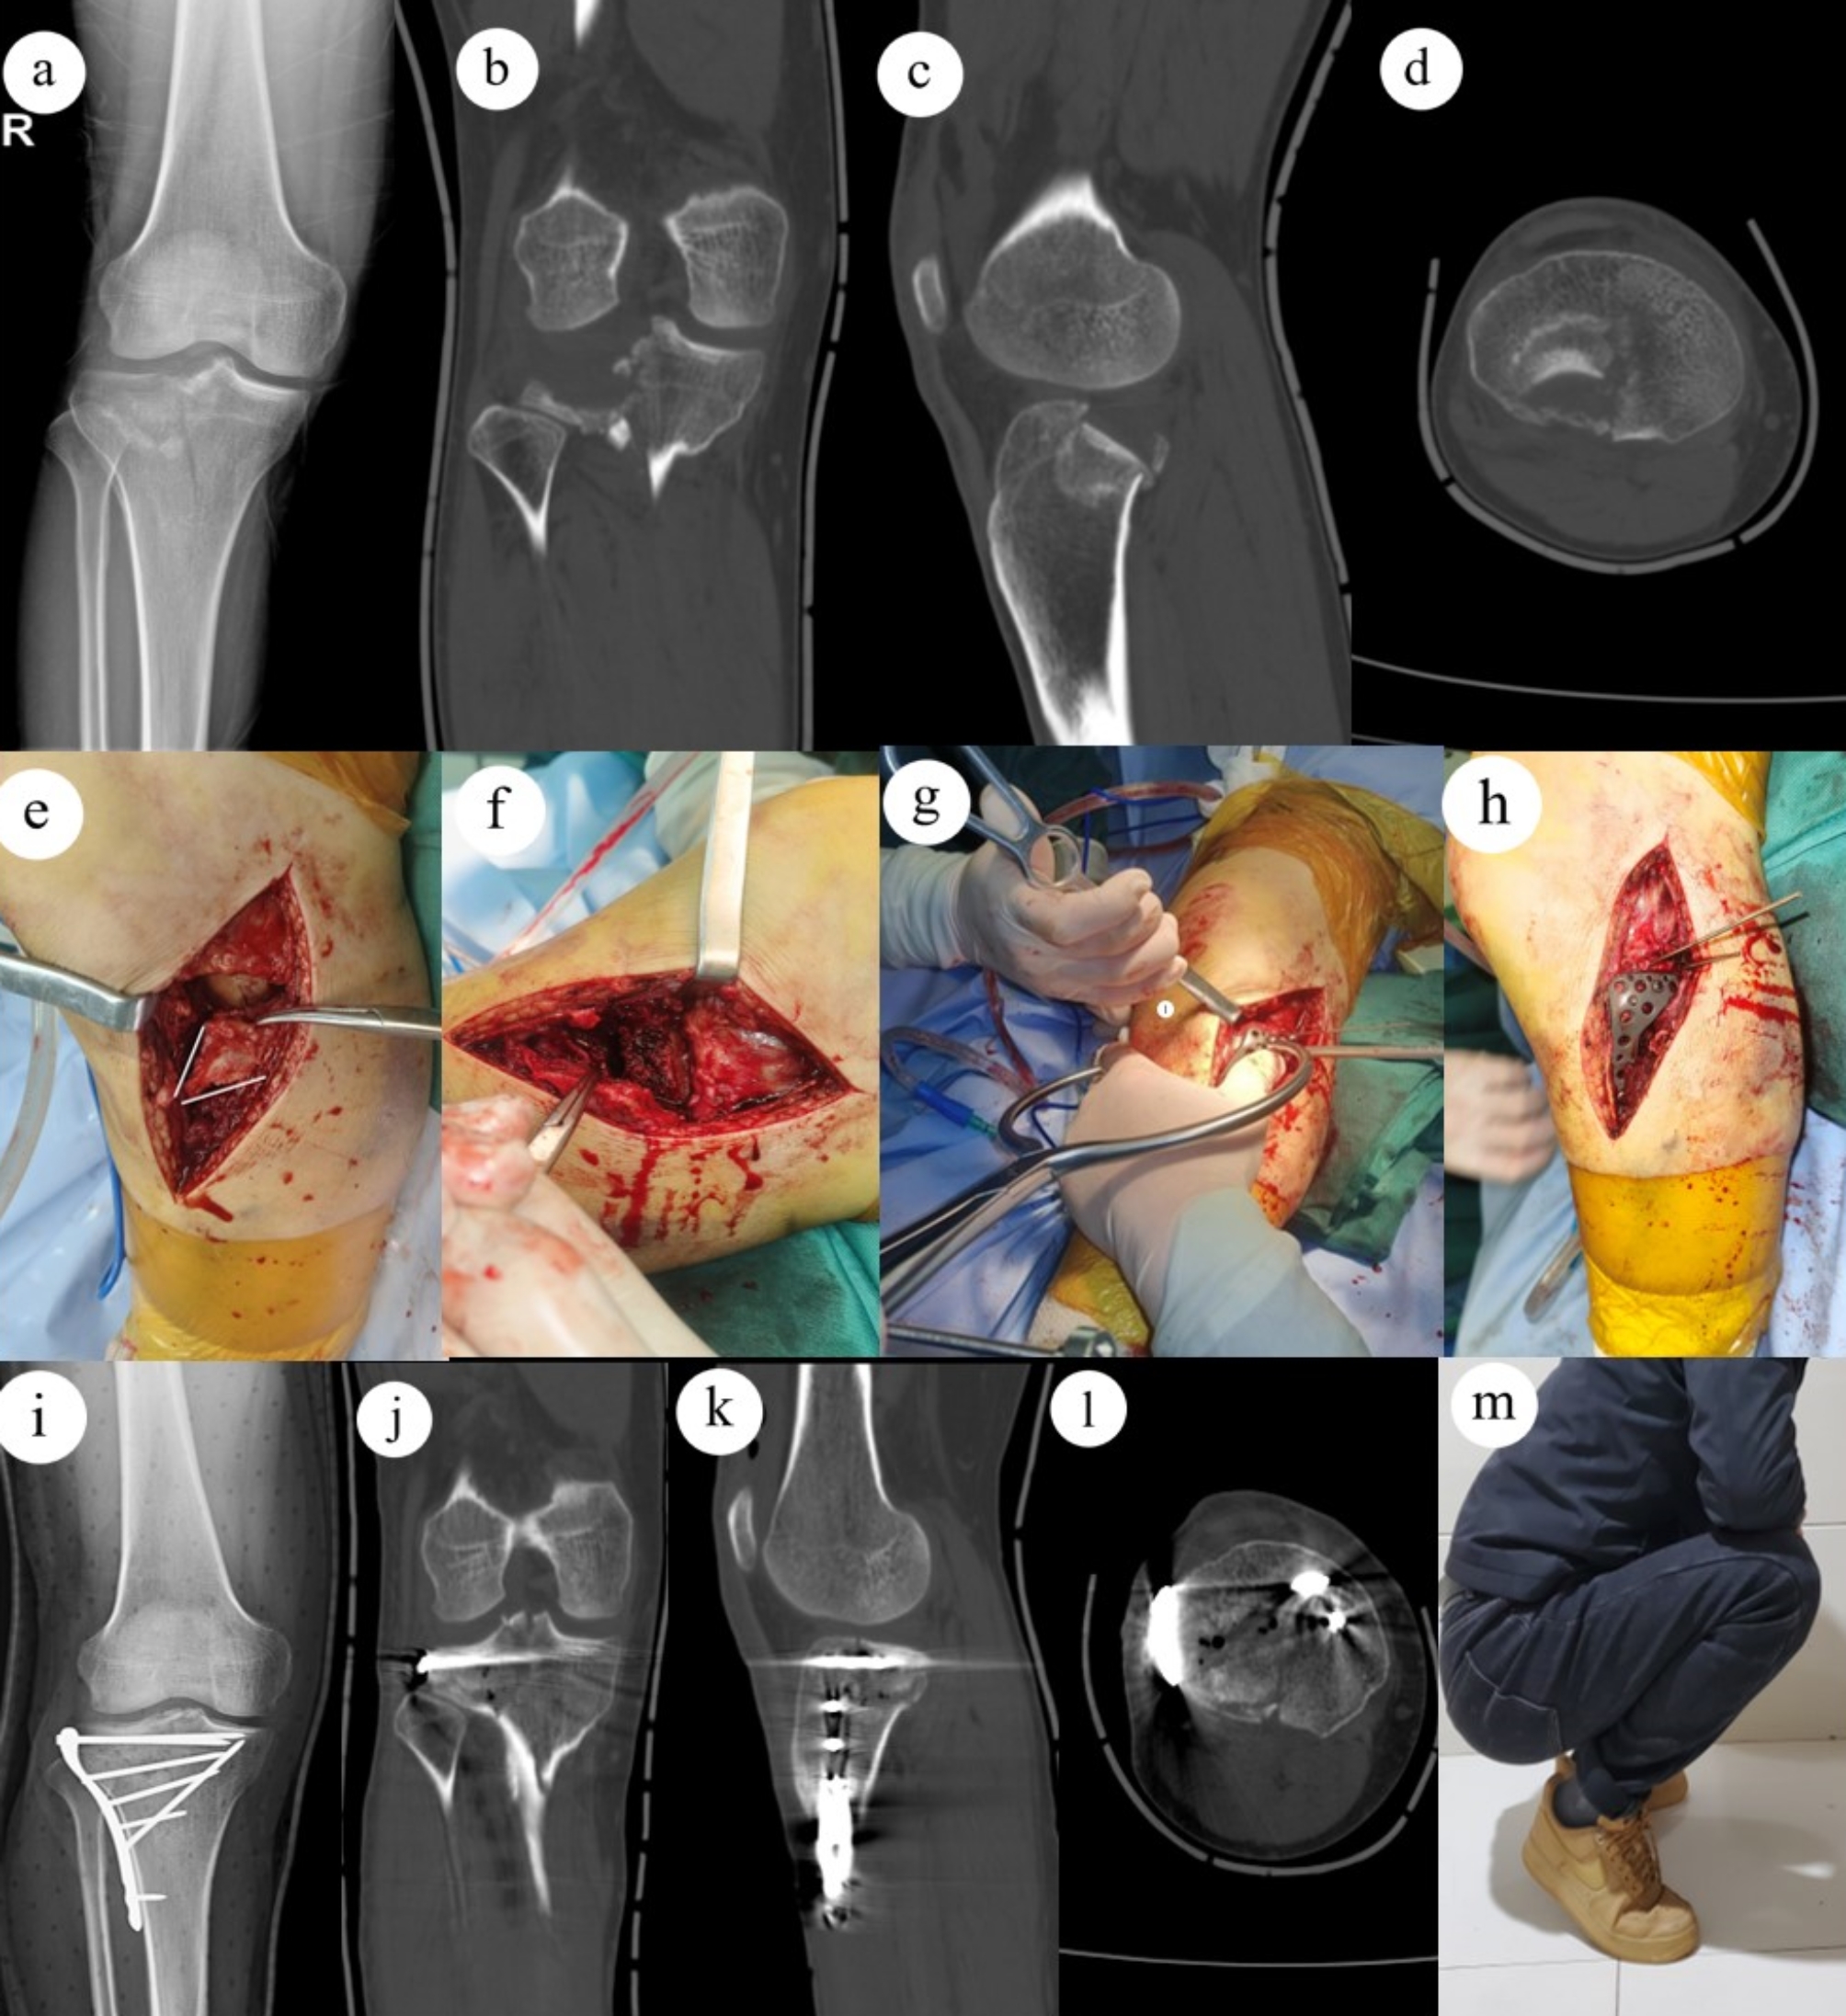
A case of type V tibial plateau fracture, male, 29 years old. a-d Preoperative X-ray and CT showed an obvious depressed posterolateral tibial plateau fracture and a splitting medial tibial plateau fracture. e The osteotomy lines are outlined by double white line. f direct visualization and manipulation of depressed posterolateral fragment were available with the external rotation of osteotomy fragment. g Intraoperative image showed the medial and lateral condyles were fixed temporarily with bone tenaculum to prevent widening of the plateau. h Intraoperative image showed a lateral locking plate was placed on the lateral rim of the tibial plateau. i-m Postoperative X-ray and CT showed that the fracture was anatomically reduced. j This patient’s 6-months follow-up
